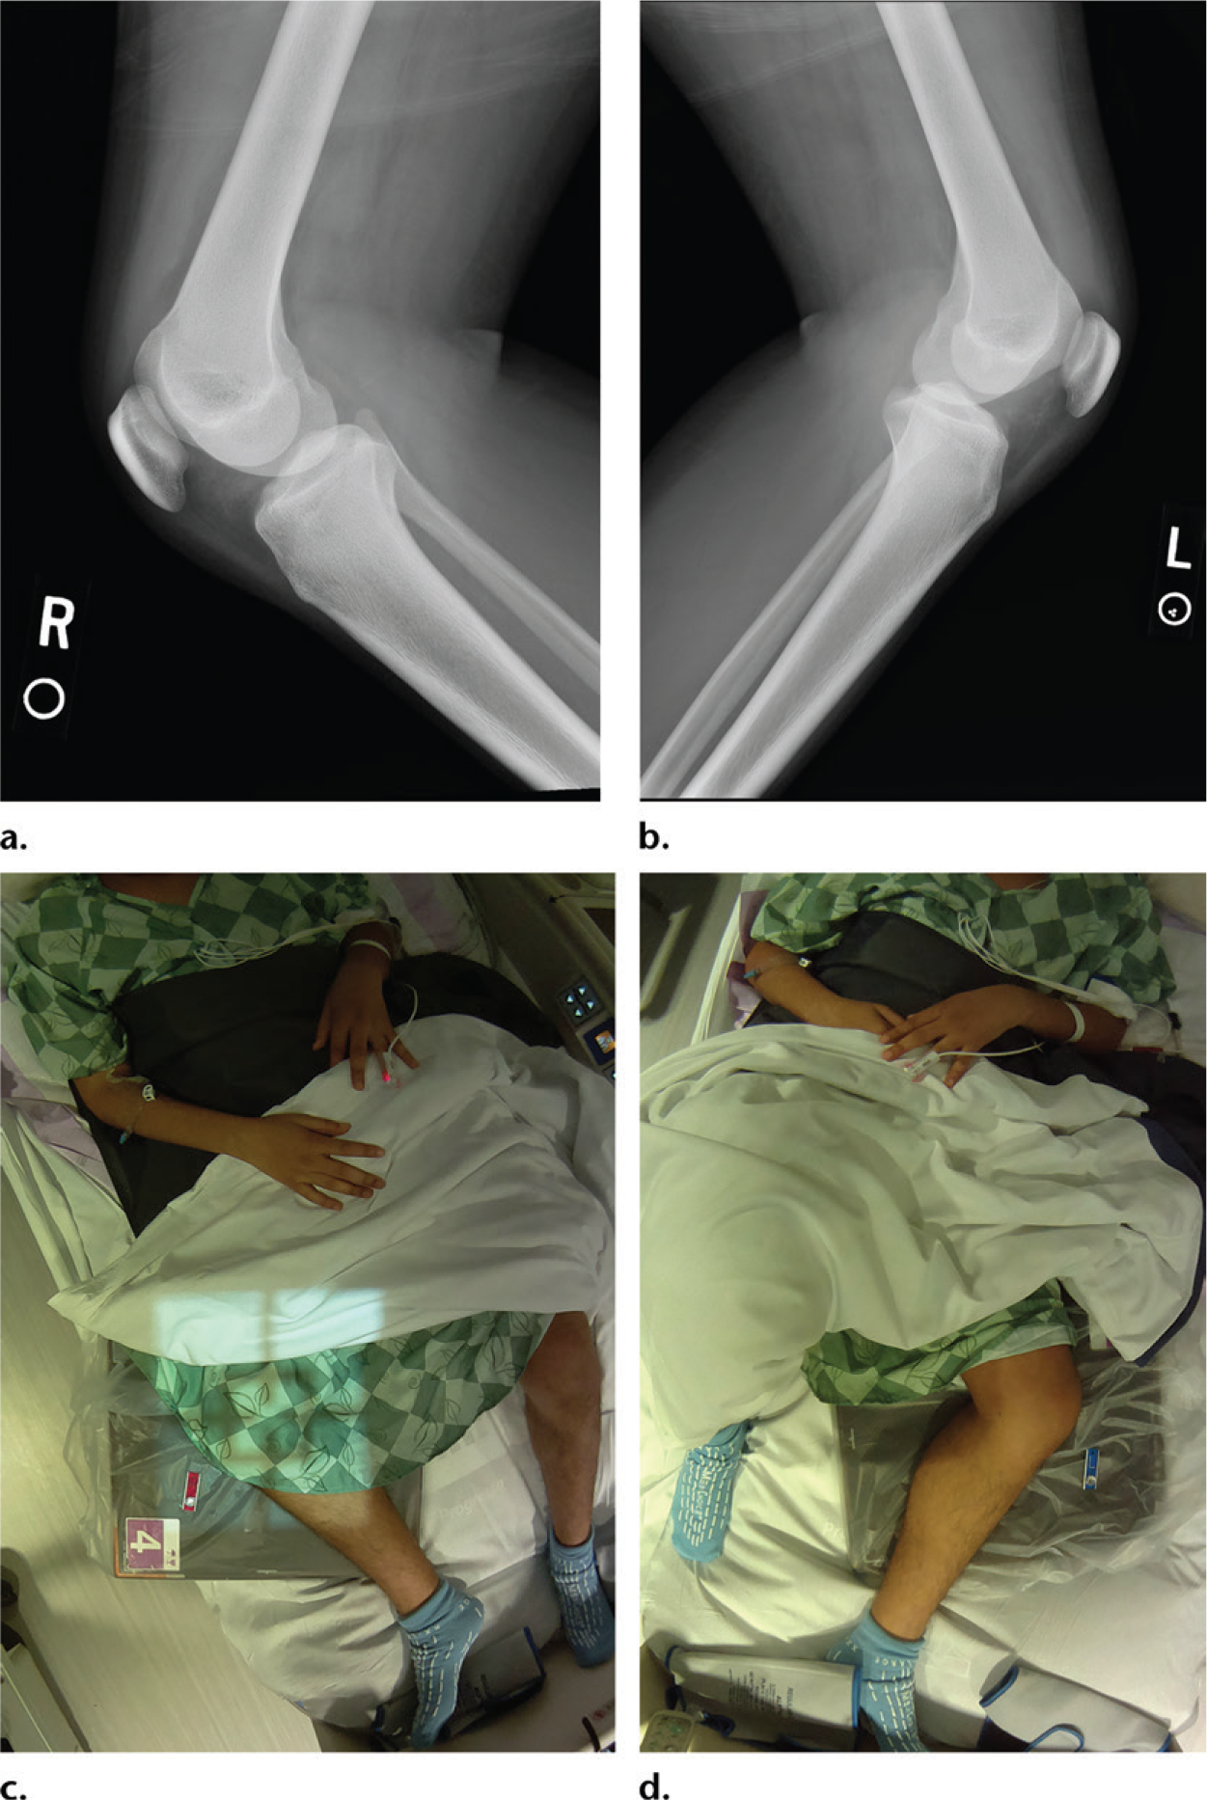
(a, b) Lateral radiographs show the right (a) and left (b) knees in a man with knee pain. (c, d) Photographs show the patient at imaging, demonstrating that the right (c) and left (d) knees were imaged. This confirms that the correct side was imaged in each case.
